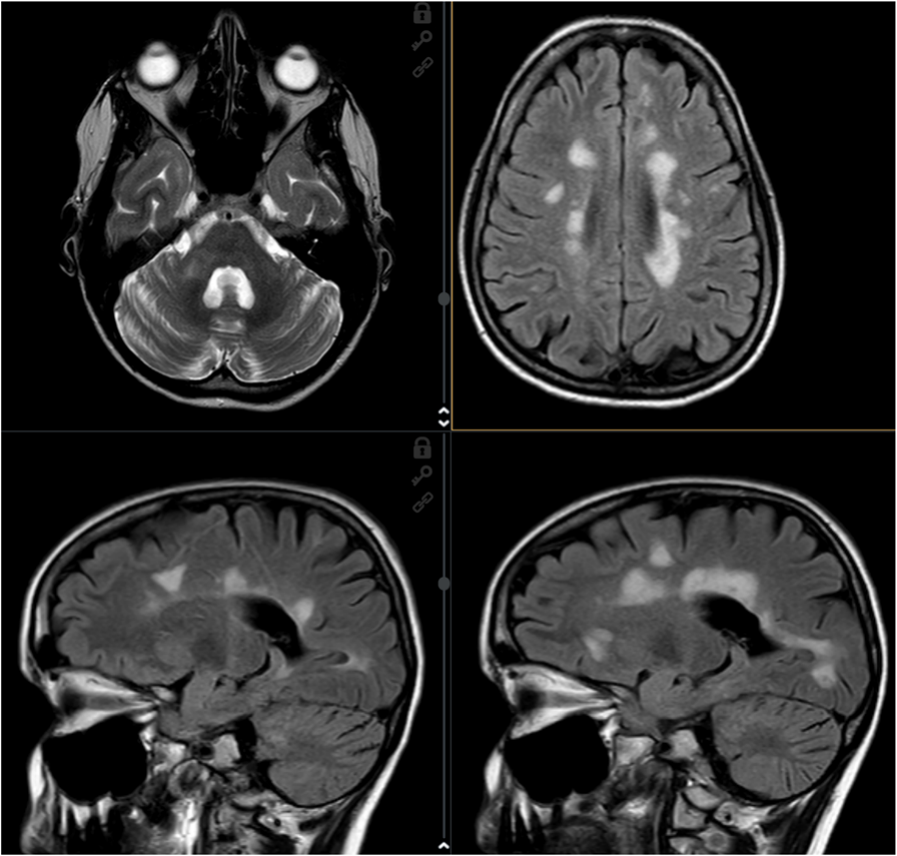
12/2019 T2 and FLAIR images. MRI after switch to ocrelizumab. Almost complete regression of inratentorial RS lesions. Supratentorial lesions without progression
